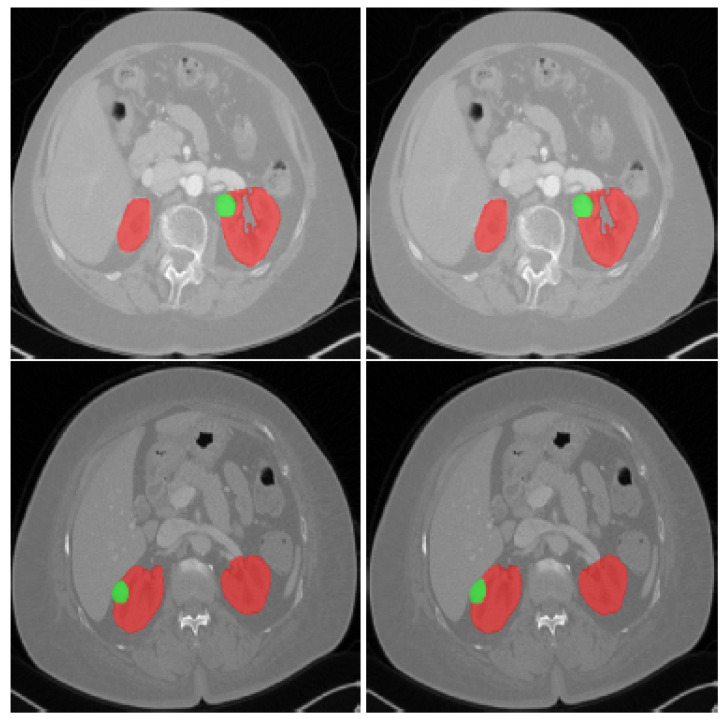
CT scans showing: An axial slice of two patients’ 3D CT scans from the KiTS19 dataset; the red tint denotes the kidneys, whereas the green color indicates the tumor site [5].
